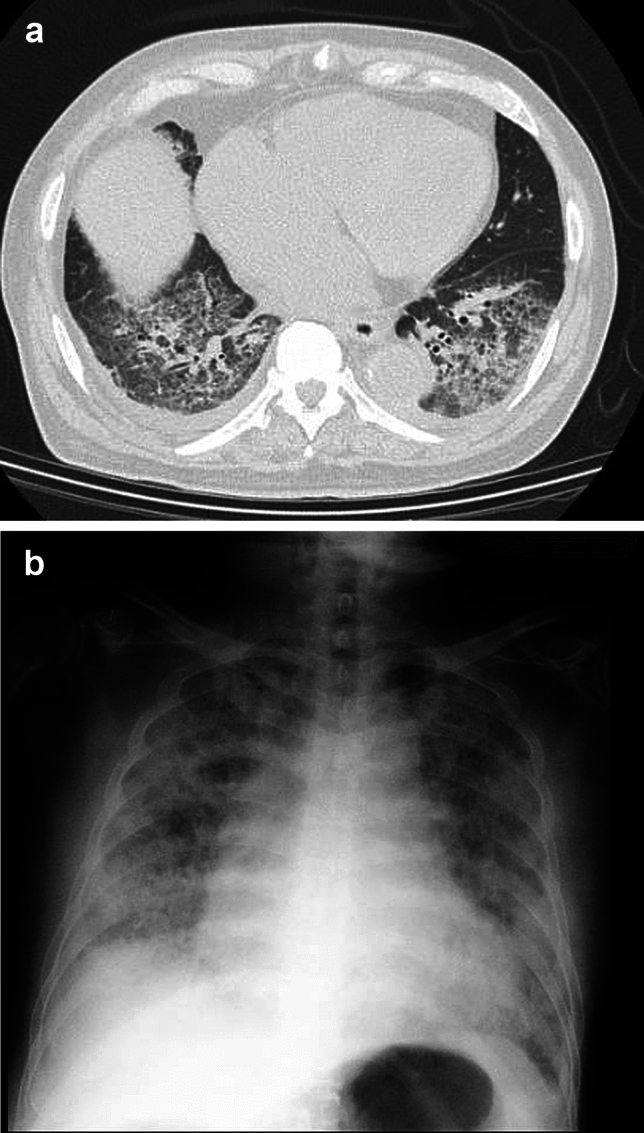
A 90-year-old male was scheduled for CT colonography as part of a detailed examination for abdominal pain. Bowel preparation included Diatrizoate meglumine and a bowel cleansing agent. On the day of the examination, the patient developed respiratory failure and was urgently hospitalized. a A chest CT scan taken on the same day revealed pneumonia in both lower lobes of the lungs, leading to a diagnosis of aspiration pneumonia caused by the bowel preparation agents. b A plain chest X-ray taken two days later showed significant worsening of the pneumonia. Despite intensive care, including steroid pulse therapy, the patient died on that day
